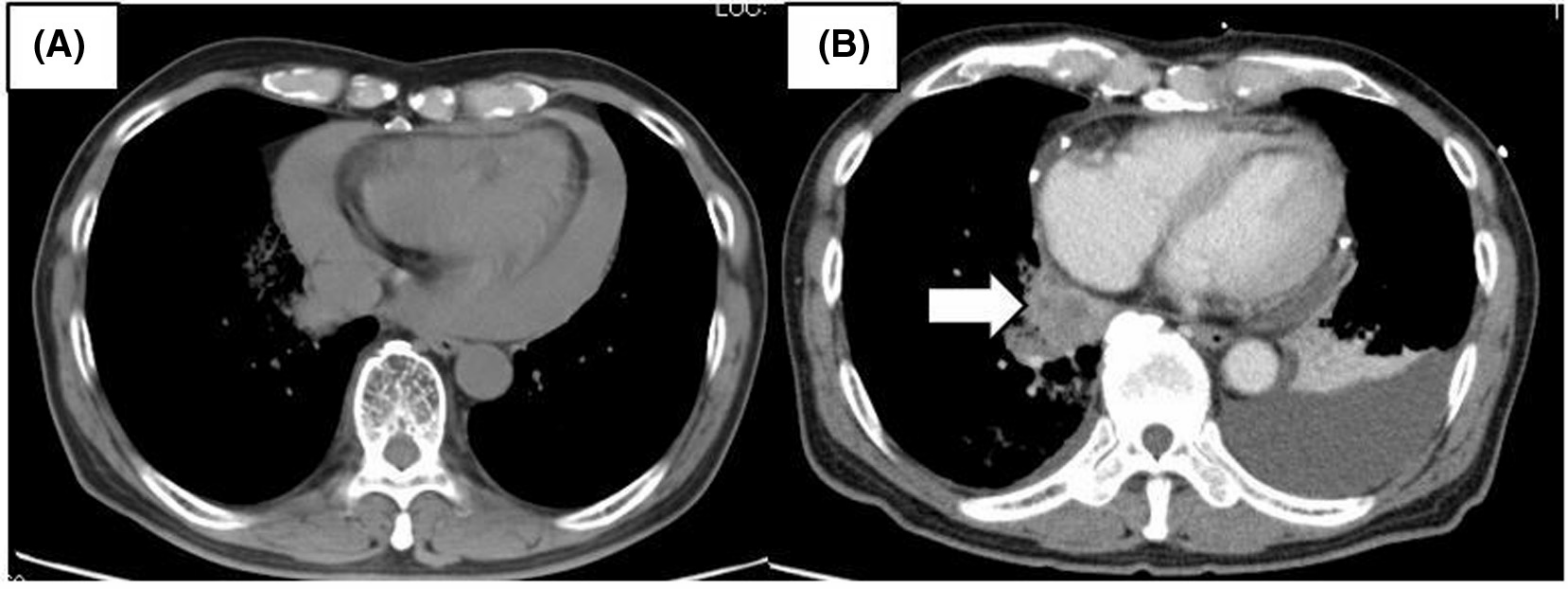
Transverse view computed tomography performed when the patient presented due to progressive dyspnea. (A) Initial chest computed tomography at Fujiyoshida Municipal Hospital showing a pericardial tamponade. Its volume was approximately 150–250 ml. (B) Computed tomography with contrast performed at our hospital showing a tumor (white arrow) and lung cancer recurrence in the right lower lobe involving the inferior pulmonary vein
